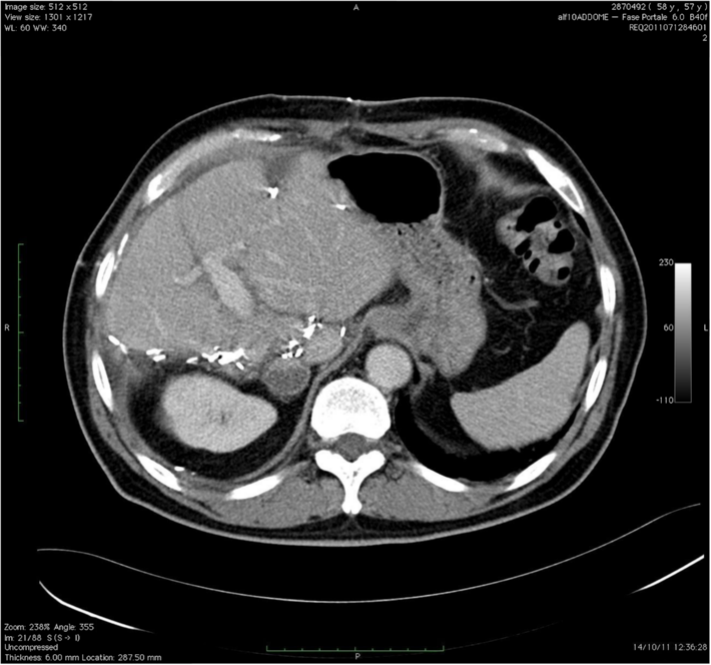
A CT scan was obtained after surgery. The outcome was unremarkable with normal liver function. The resected area is highlighted by the hemostatic clips left *in situ*. CT, computed tomography.
